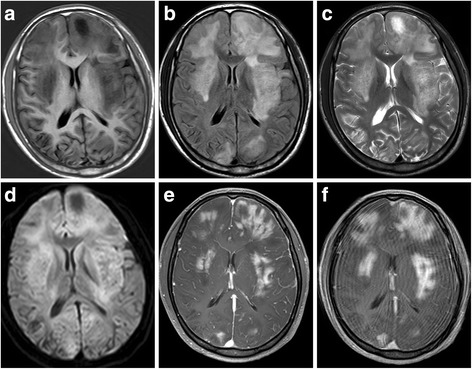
Twenty one-year-old man with lymphomatosis cerebri. a, b and c, Axial T1WI,T2-weighted FLAIR and T2WI show the distribution of type I and II lesions, which involved bilateral frontal lobes, occipital lobes, basal ganglia and insula. d DWI shows slight hyperintensity in the lesions, which were also slightly hyperintense on ADC map(not shown). e Post-contrast T1WI shows multiple patchy contrast enhancement in the lesions. f, Delayed scanning of post-contrast T1WI shows extended enhancement range of the lesions
